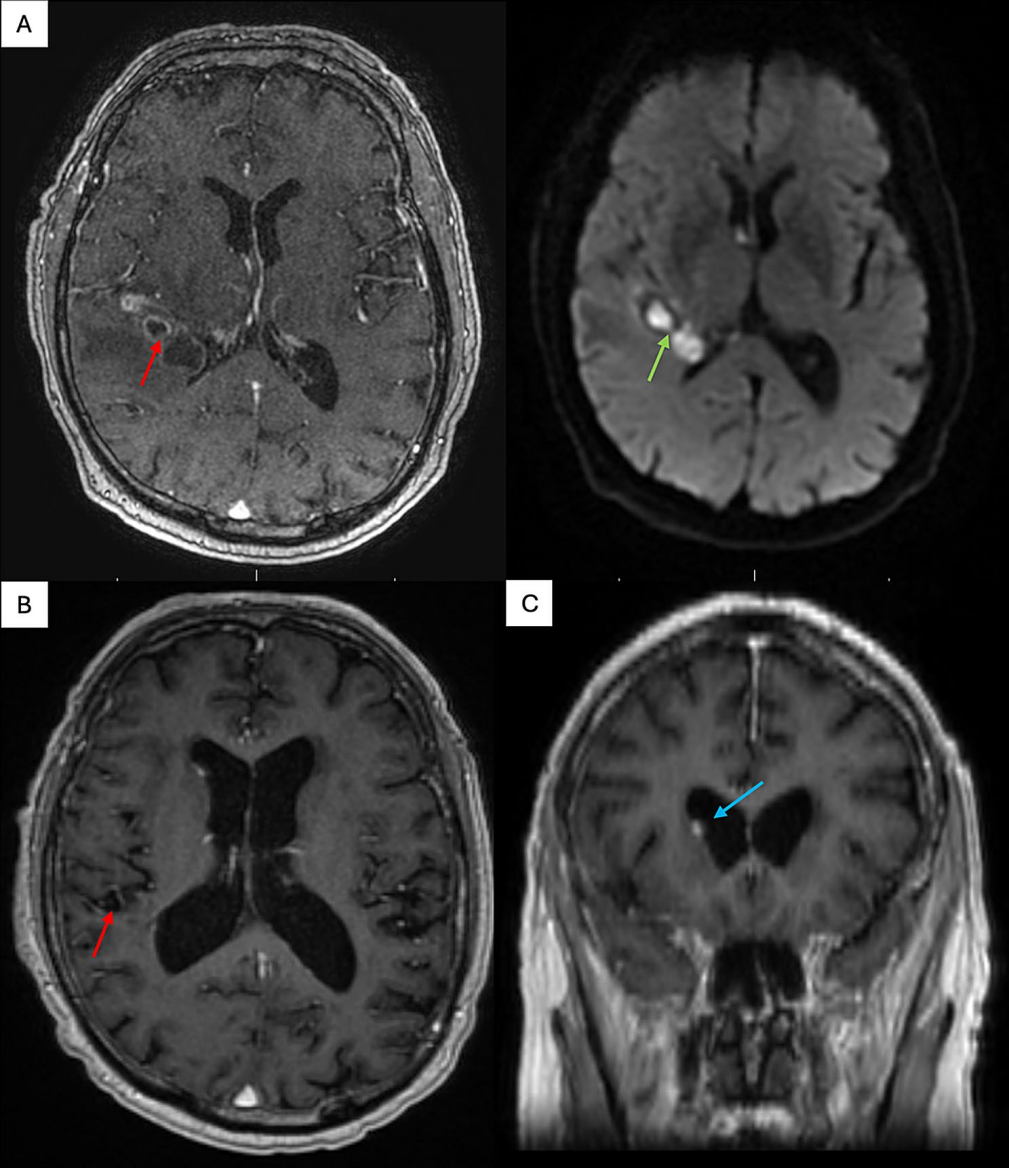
Preoperative MRI imaging. A: T1 weighed axial MRI sequence with contrast depicting multiple abscess-suspicious lesions adjacent to the posterior horn of the right lateral ventricle with marginal contrast enhancement (red arrow) and DWI axial sequence with characteristic diffusion restriction (green arrow). B: T1 weighed axial MRI sequence with contrast depicting significant reduction in abscess formation after surgical burr hole evacuation (red arrow). C: T1 weighed coronal MRI sequence with contrast depicting small ependymal lesion adjacent to the lateral wall of the right lateral ventricle (blue arrow). MRI = Magnetic Resonance Imaging; DWI = Diffusion Weight Imaging
